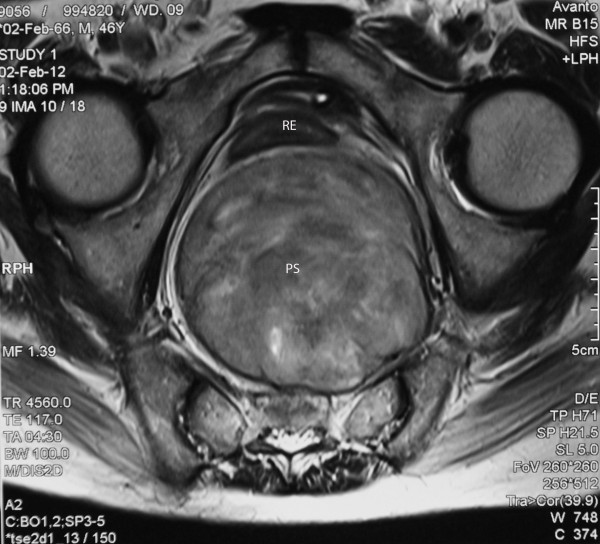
Magnetic resonance imaging of the presacral pelvic (PS) compressing the rectum (RE); transverse section.
